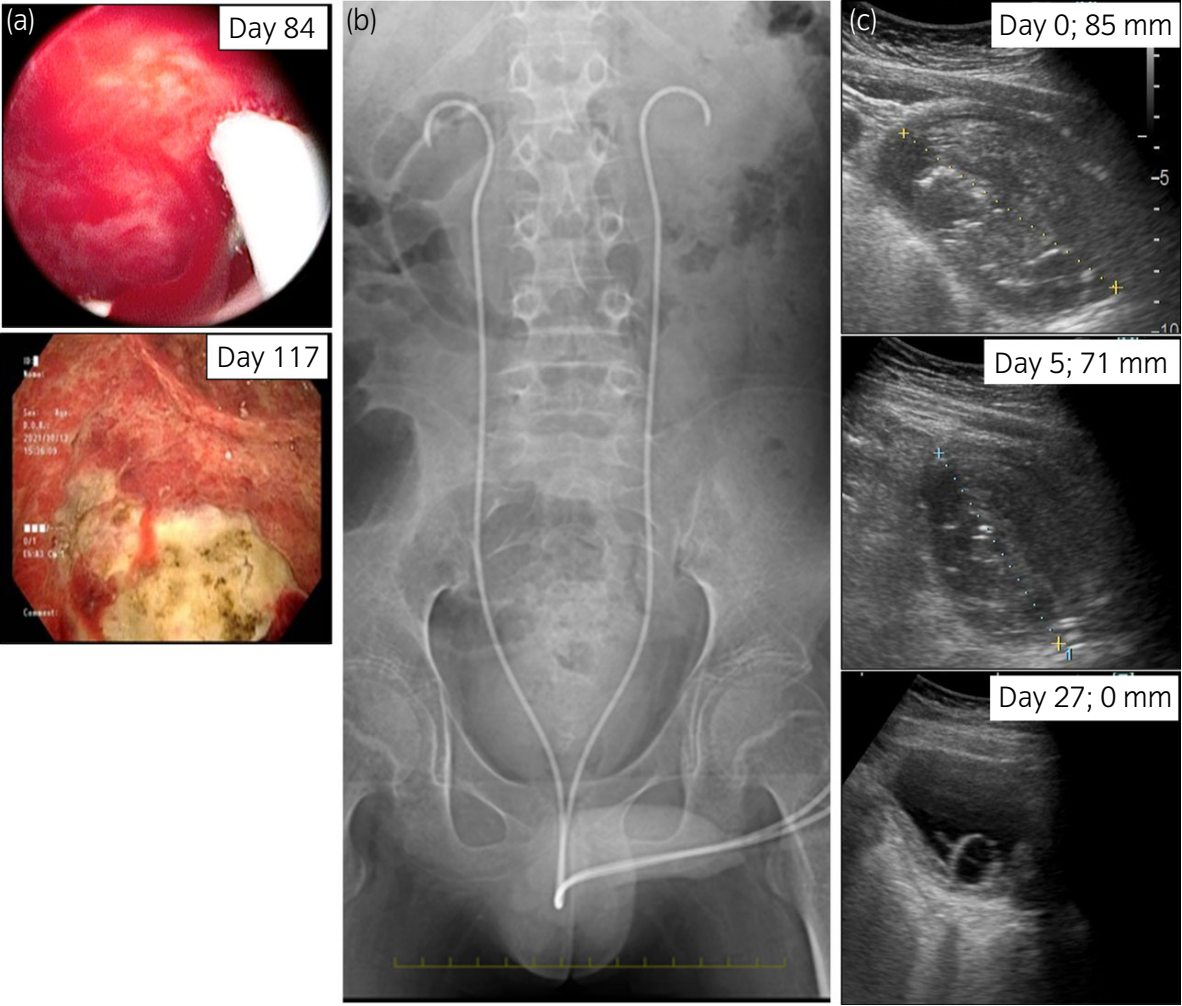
Urologic interventions and the change of the bladder blood clot. (a) Images of the bladder wall during TUE with cystoscope on day 84 (upper panel) and day 117 (lower panel) after HSCT. (b) X‐ray image taken during bilateral single‐J ureteral stent placement. (c) Sagittal section on ultrasonography of the bladder blood clot on days 0, 5, and 27 post‐stenting. The long diameter of the clot was reduced from 85 to 71 mm and then to 0 mm. [Colour figure can be viewed at wileyonlinelibrary.com]
